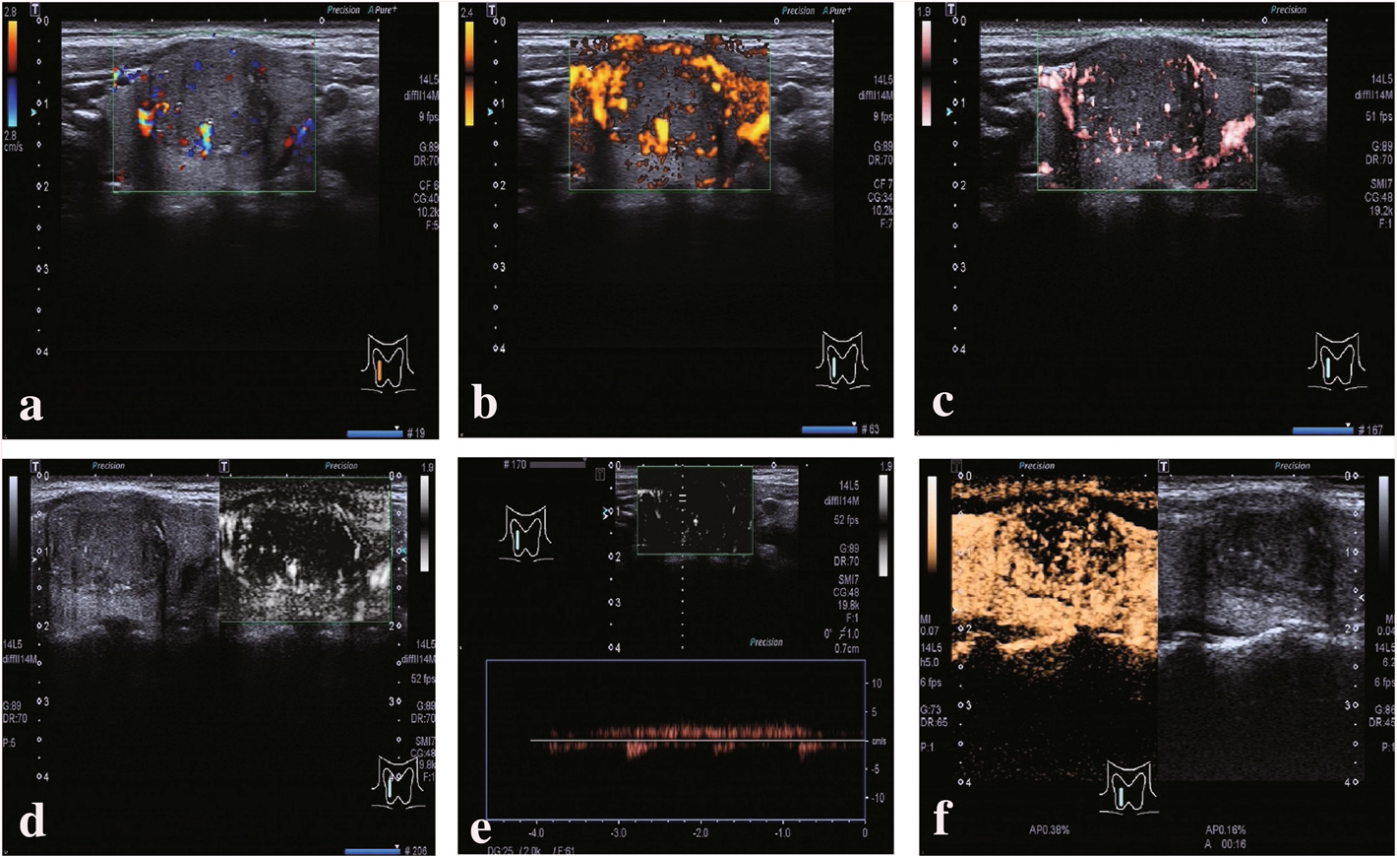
A papillary thyroid carcinoma located in the right lobe. a CDI and b PDI show peripheral and internal vascularity of the nodule, with c color SMI and d monochrome SMI depicting more detailed peripheral vascularity surrounding the nodule as well as internal disordered small branching microvessels. e Pulsed Doppler (guided by monochrome SMI) established that the small branching microvasculature was real and measurable. f CEUS features of the nodule. The nodule enhances later compared with normal thyroid parenchyma, with disordered heterogeneous hypoechoic enhancement and incomplete peripheral enhancement
